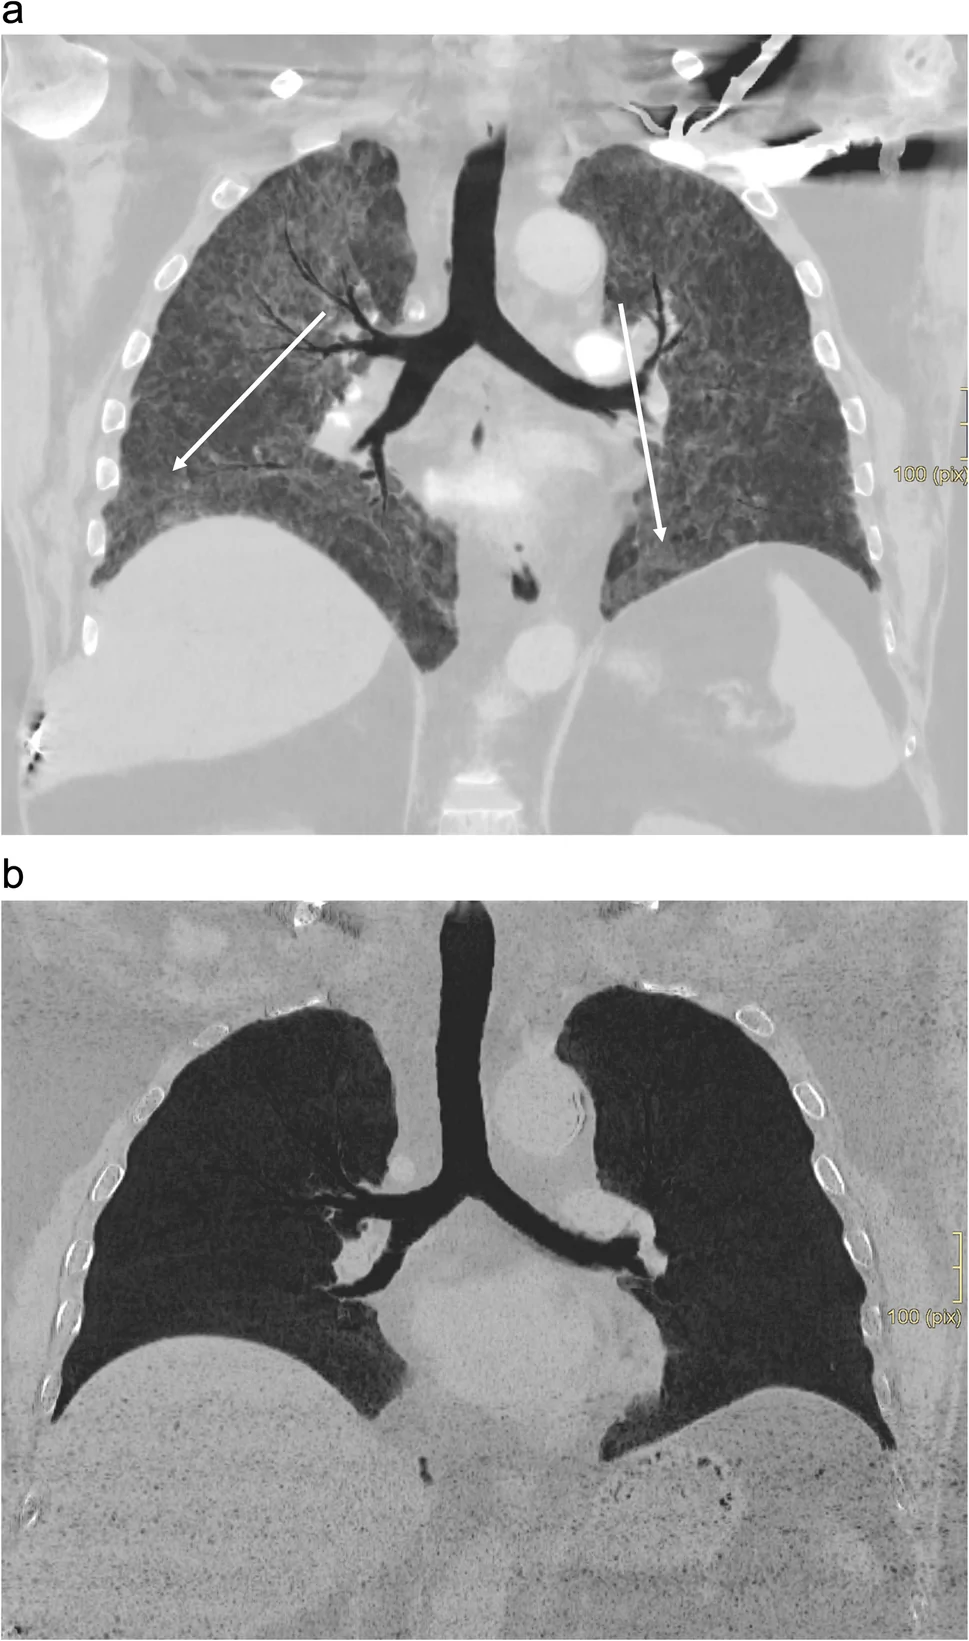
Chest CT at diagnosis and after treatment a Coronal CT image at diagnosis showing bilateral ground-glass opacities and intralobular reticulations, highlighted by arrows b One-month follow-up CT showing near-complete resolution after pomalidomide withdrawal and systemic corticosteroid therapy
